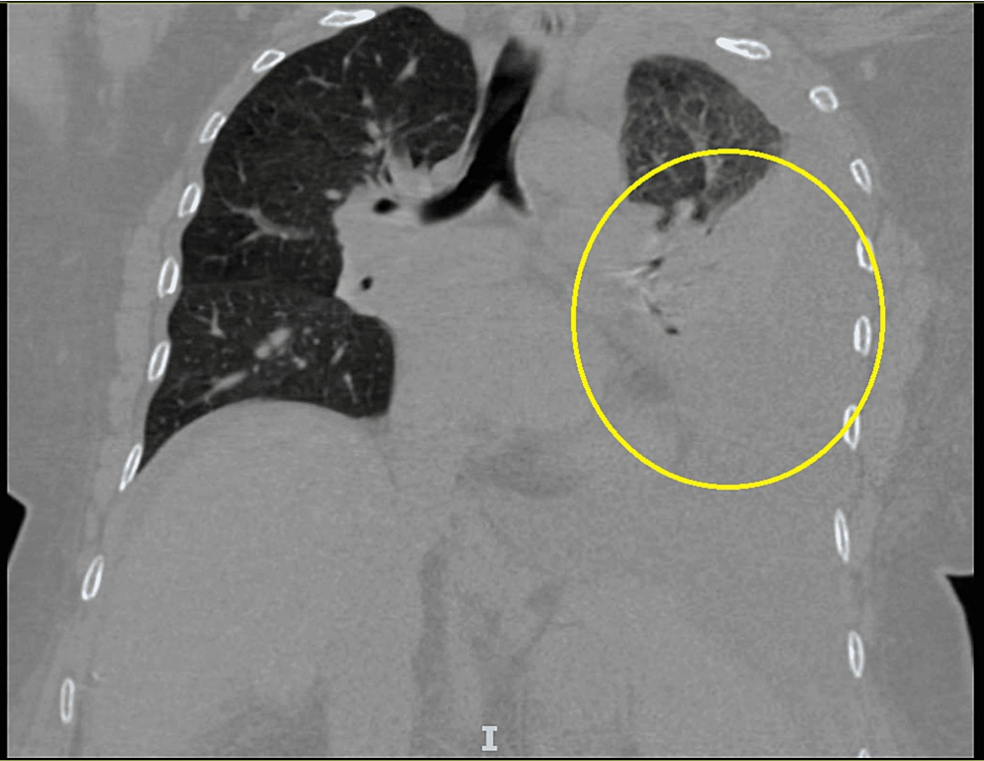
CT Chest Yellow circle: pleural effusion
